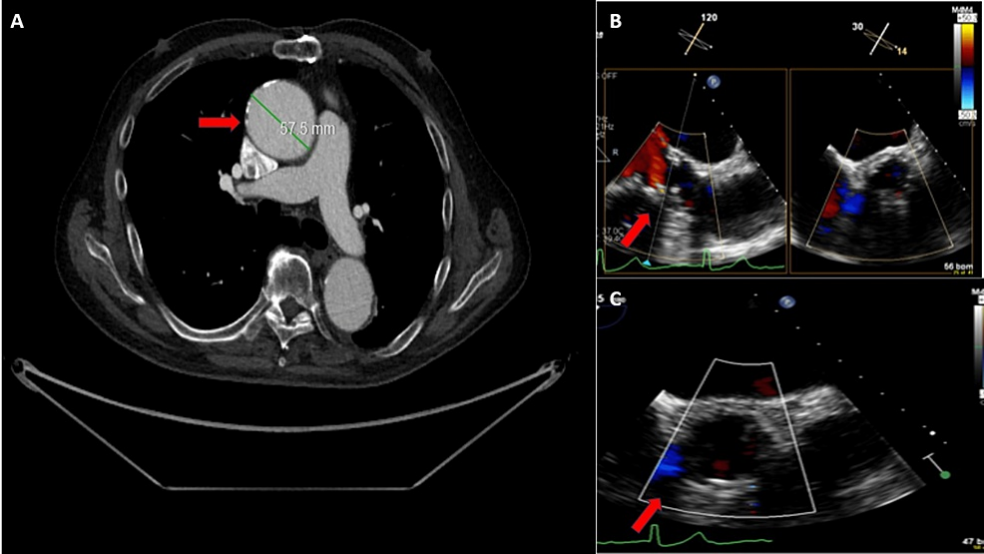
A. Post-op CTA showing slight improvement in AAA (red arrow). B. Post-op TEE showing significant improvement in the PVL (red arrow). C. Three-month follow-up TEE showing trace PVL (red arrow). CTA, computed tomography angiography; TEE, transesophageal echocardiogram; PVL, paravalvular leak; AAA, abdominal aortic aneurysm
